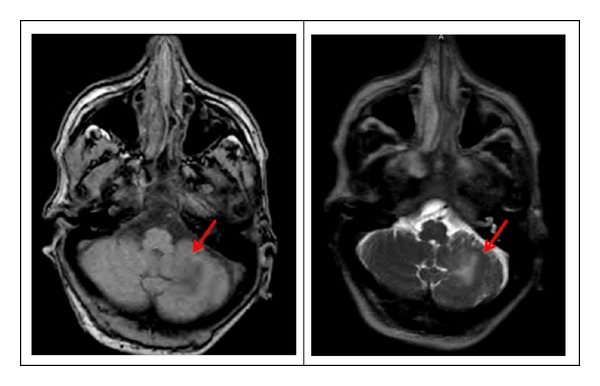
MRI of the brain revealing a region of hyperintense T2 signal in the left inferior cerebellar hemisphere involving the cortex and underlying white matter (shown by arrows). The area of involvement measures 2 cm approximately. No abnormal enhancement was shown after the administration of gadolinium.
